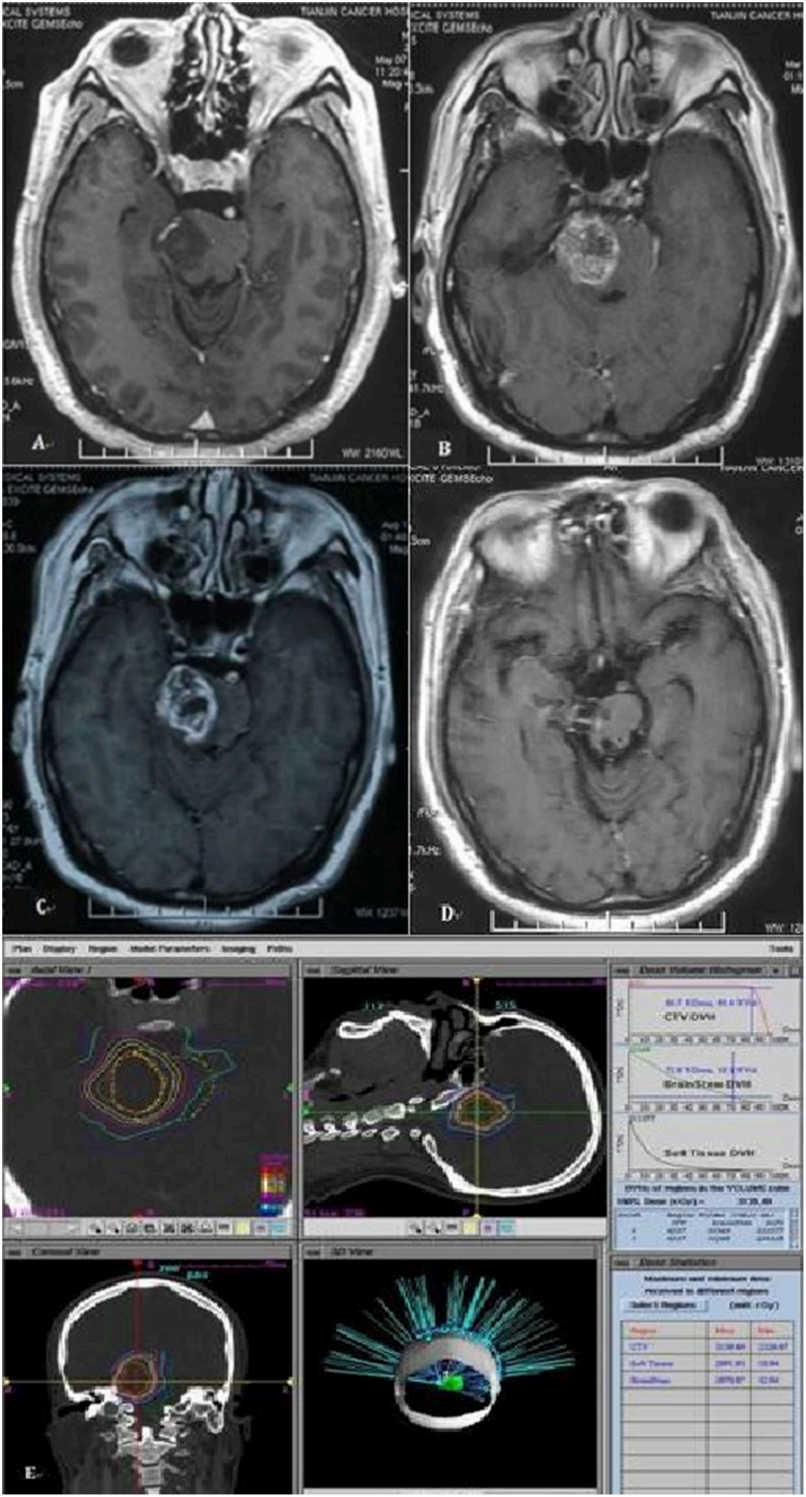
Magnetic resonance image of patient with pseudoprogression. (A) MRI of a 45-year-old man with pons glioma diagnosed by MR. (B) Eleven months after treatment, pseudoprogression happened on MR. (C) Fifteen months after treatment. (D) Two year after treatment, pseudoprogression gradually resolution. (E) The ROI volume of this patient is 8.2 ml with 2,700 cGy.
